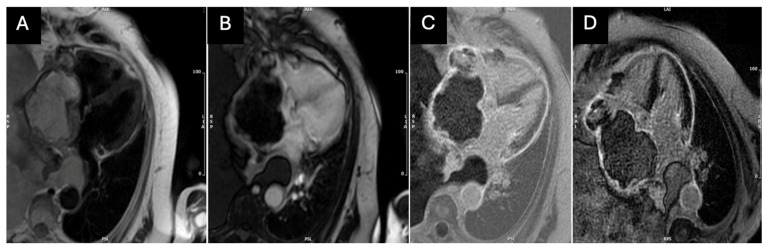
Four-month follow-up CMR imaging showing right-sided mass (A) in black blood T2-weighted image long-axis view heterogenous signal; (B) early gadolinium enhancement; (C) phase-sensitive inversion recovery (PSIR) late gadolinium enhancement (LGE); and (D) magnitude IR late gadolinium enhancement (LGE) heterogeneous uptake of contrast.
